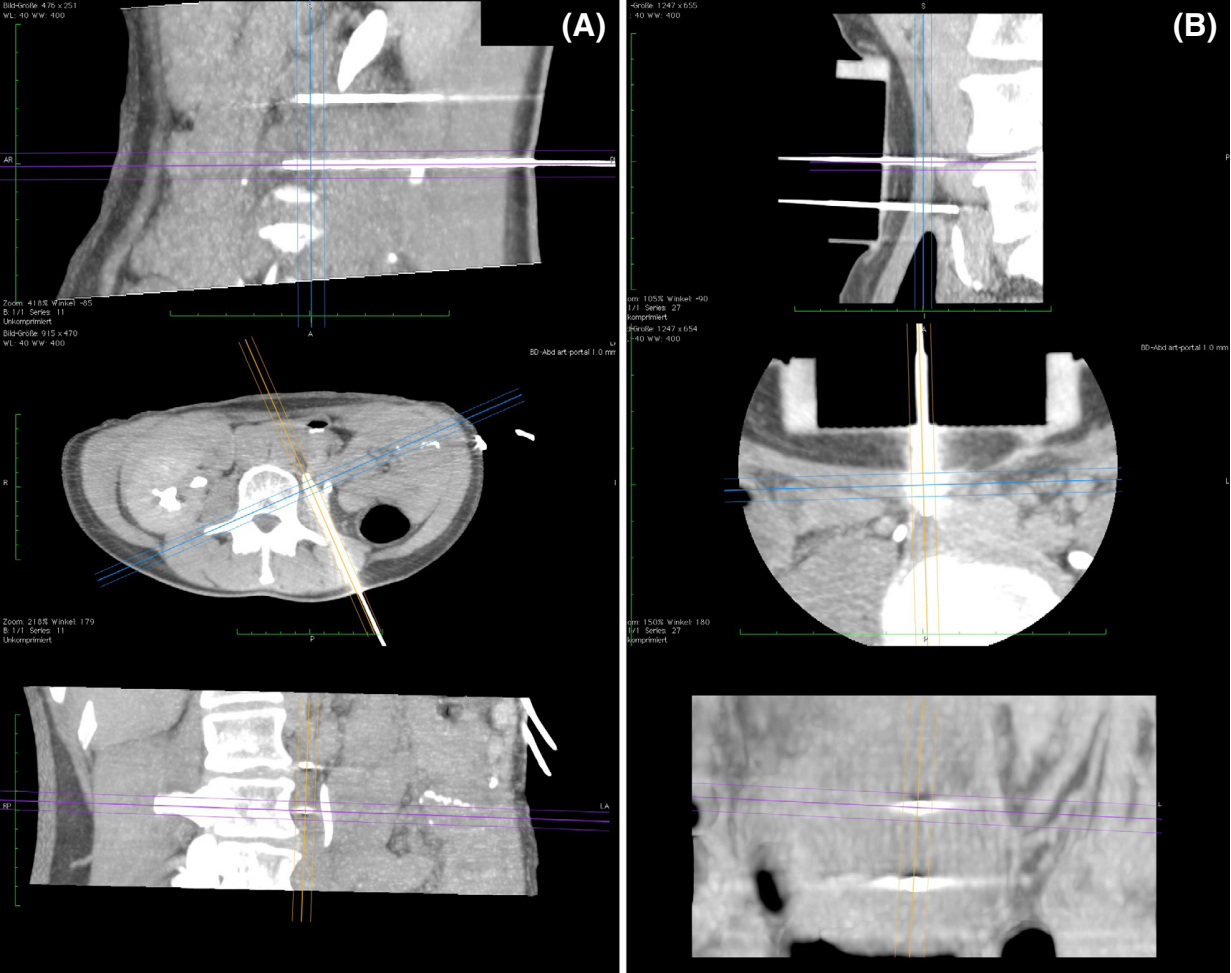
CT images of the patient during the intervention, showing the positioning of the electrodes. A. Posterior approach: Percutaneous insertion of the electrodes through the paraspinal musculature and the psoas muscle for the treatment of a metastatic lymph node located para‐aortal. B. Anterior approach through the lower abdominal wall: Treatment of the second metastatic lymph node, located pre‐caval, with the patient lying in the supine position.
